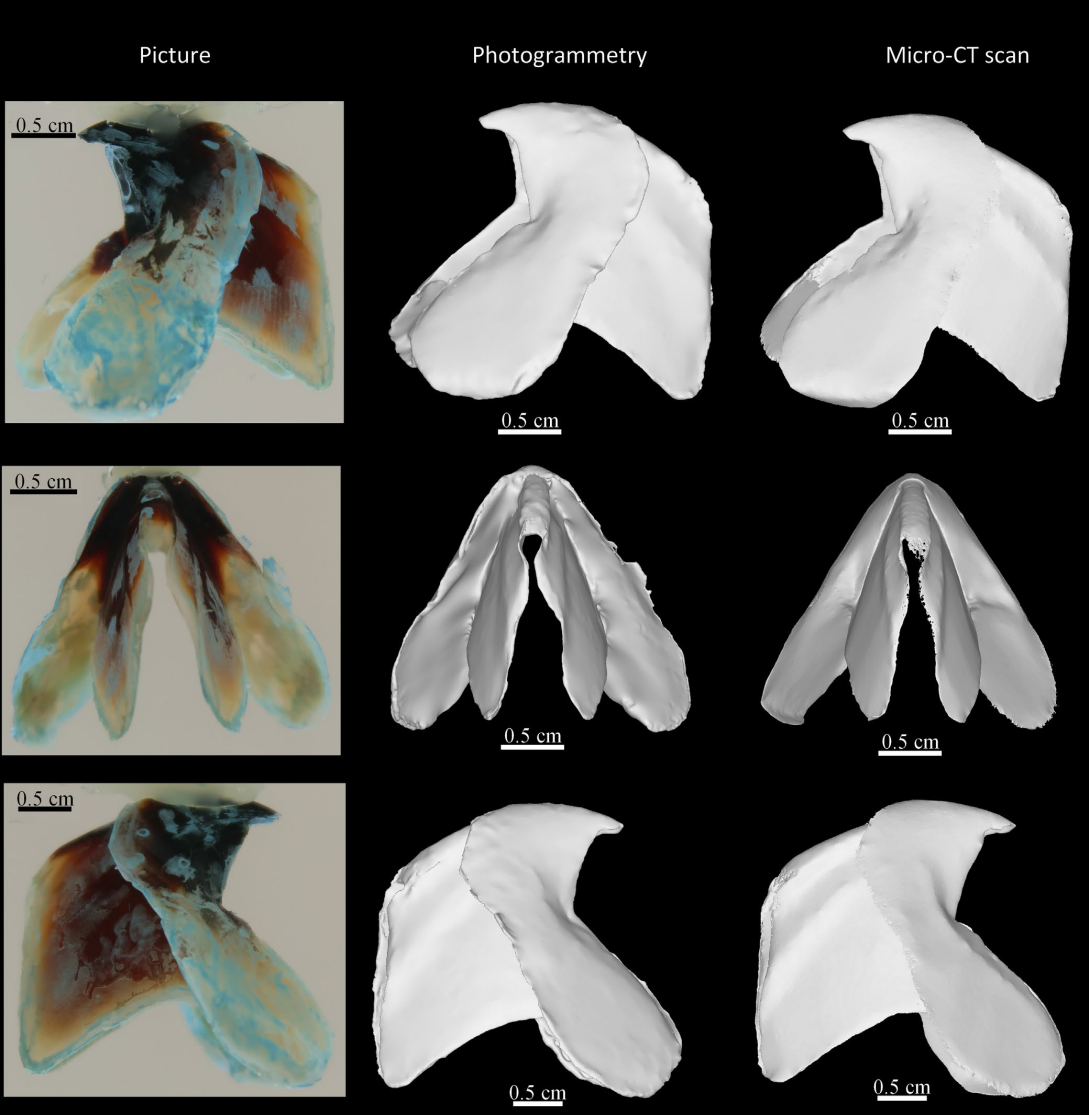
Comparison between photogrammetric model of a lower beak of *Ommastrephes cylindraceus* and the same reconstruction using micro‐CT scanning. top: lateral right view; middle: posterior view; bottom: lateral left view. Pictures conserve the conventional orientation of beaks
